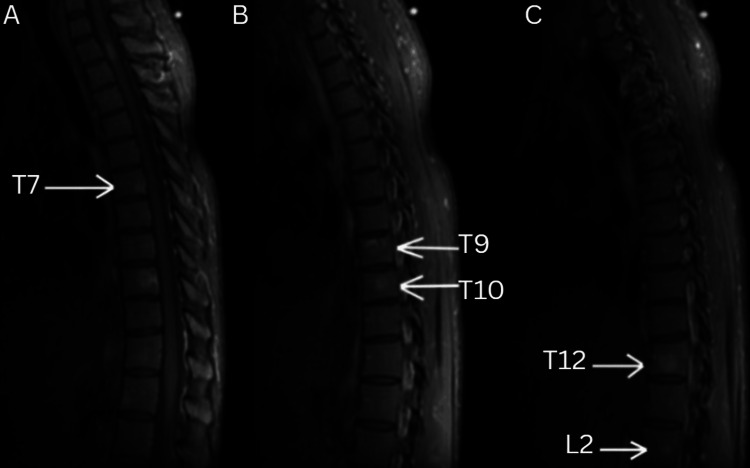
Sagittal T1 non-contrast MRI (A) Sagittal T1 non-contrast MRI demonstrating metastatic lesions within the T7 vertebral body (arrow). (B) Sagittal T1 non-contrast MRI showing additional vertebral body lesions at T9 and T10 (arrows). (C) Sagittal T1 non-contrast MRI of the thoracolumbar junction demonstrating metastatic lesions within the T12 and L2 vertebral bodies (arrows). Abbreviation: MRI, magnetic resonance imaging.
